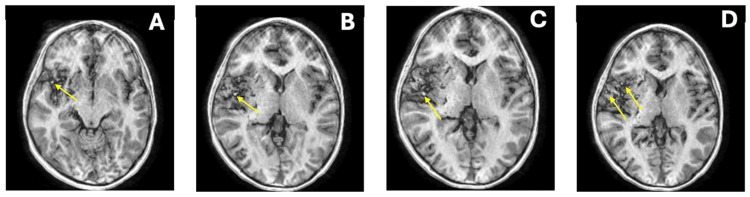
Axial T1-weighted MRI images (A-D) demonstrating a large vascular malformation in the right cerebral hemisphere (yellow arrows), with associated mass effect and partially empty sella
